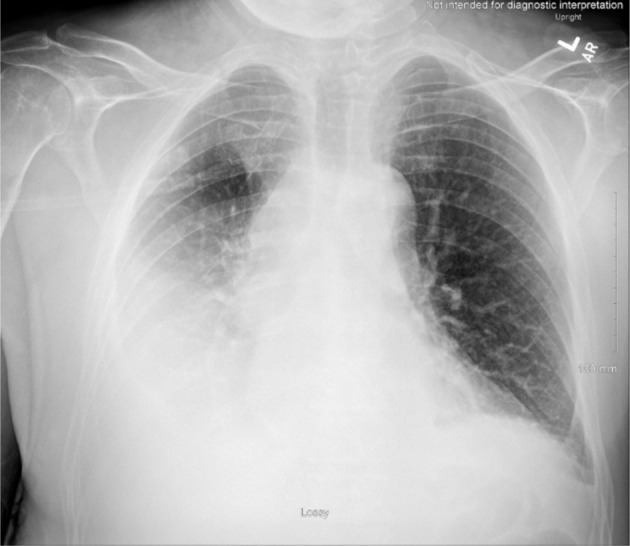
Chest X-ray obtained on hospital day 14, depicting an increase of right pleural effusion. Trace left pleural effusion and bibasilar areas of consolidation that may reflect atelectasis.
